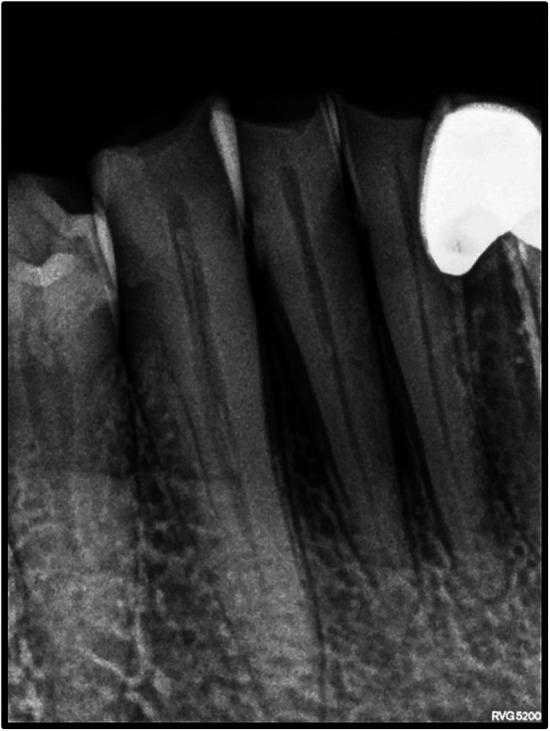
Intraoral periapical radiograph showing 41, 42, and 43 with mild horizontal bone loss.
